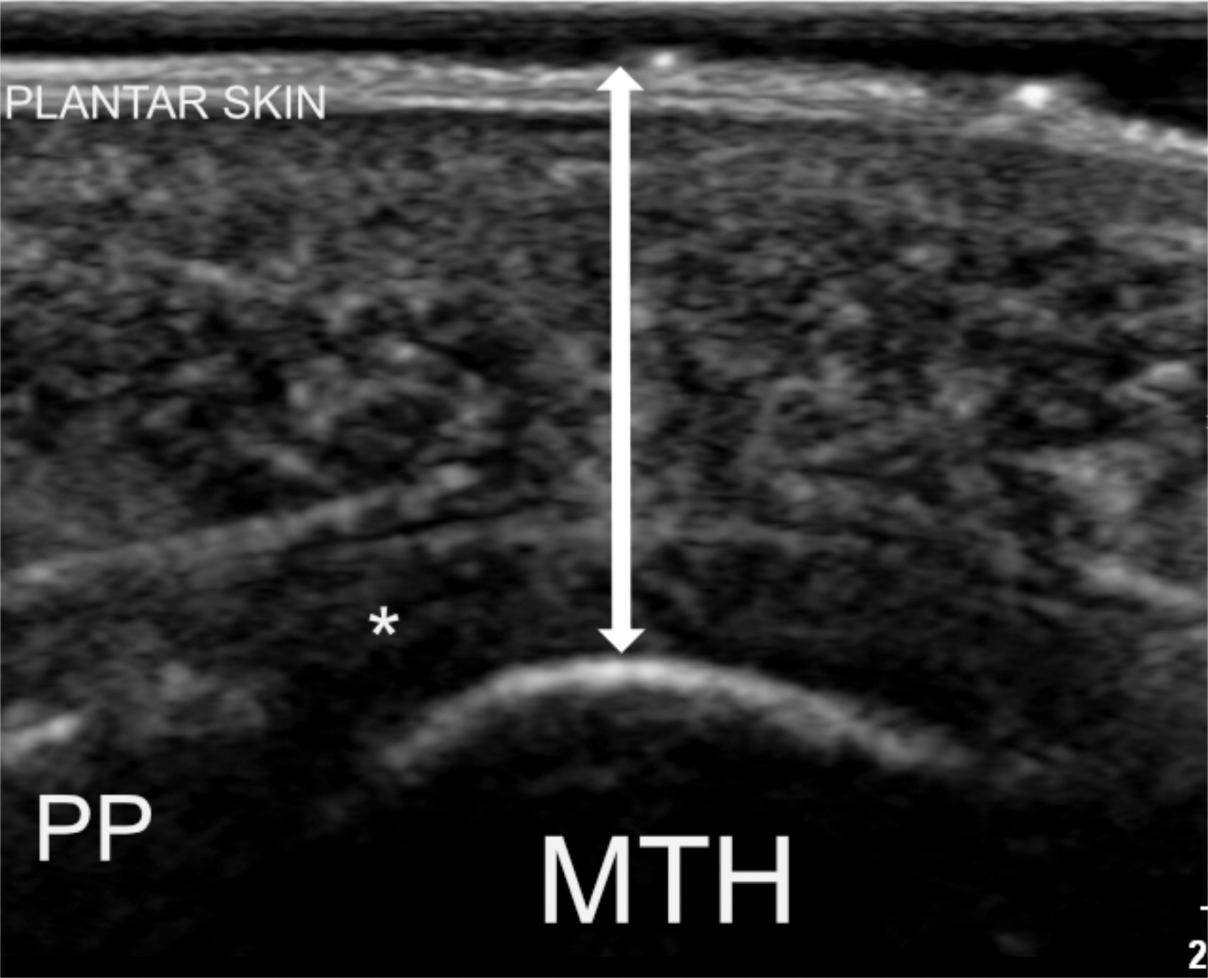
Longitudinal ultrasound image overlying the second metatarsal head demonstrating transducer positioning and measurement location (line with arrows). MTH; metatarsal head, PP; proximal phalanx, asterisk; plantar plate.
